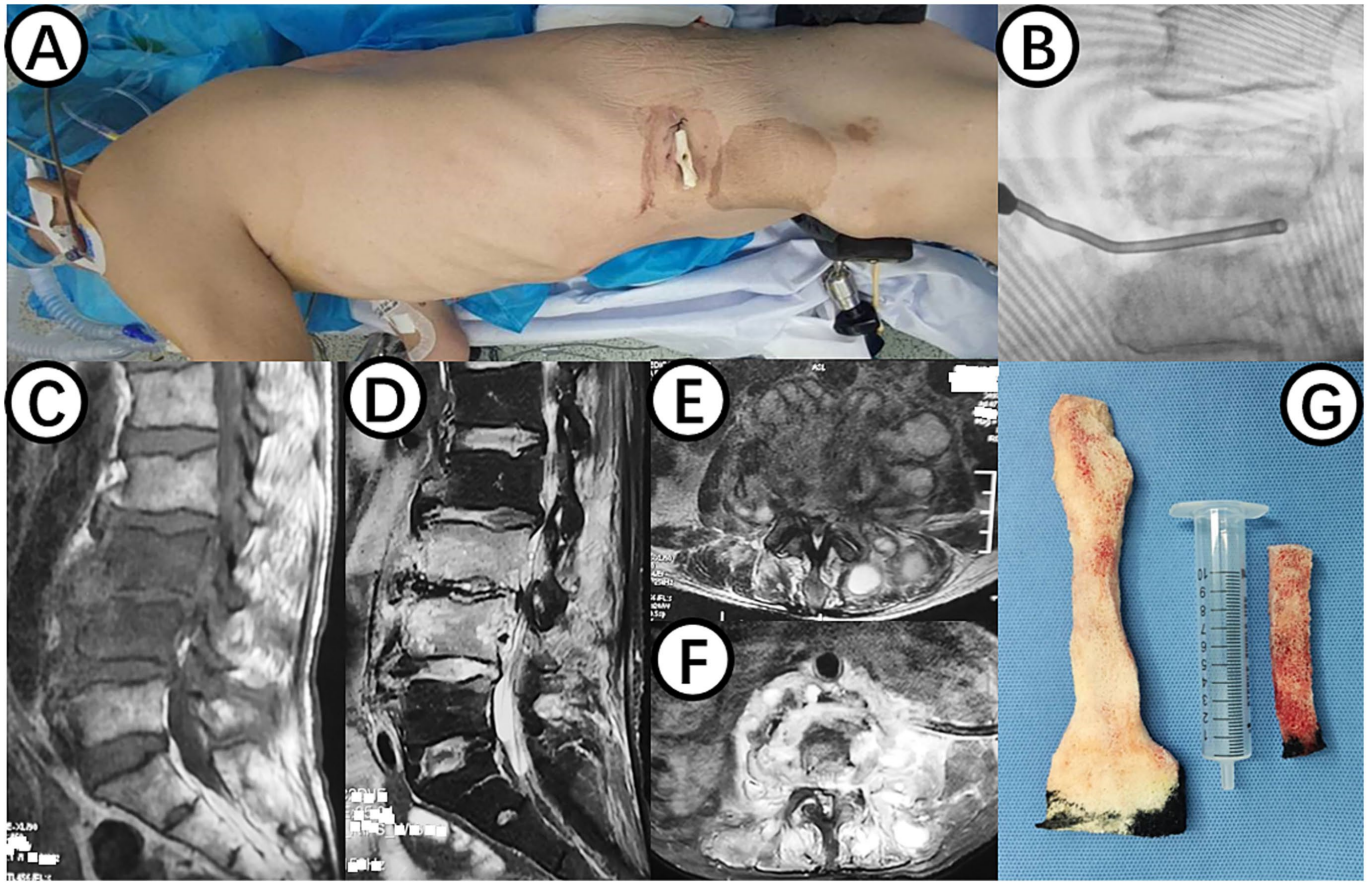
(A) Shows the surgical position for changing NPWT. (B) Displays intraoperative C-arm fluoroscopy positioning. (C,E) Present preoperative MRI scans showing high signal in the L3 and L4 vertebral bodies and intervertebral space, as well as abscess in the intervertebral space, bilateral lumbar muscles, and paraspinal muscles. (D,F) Demonstrate postoperative MRI showing decreased inflammation and edema signals in the L3 and L4 vertebral bodies and intervertebral space, with negative pressure sponge and drainage tube in place. (G) Depicts the NPWT sponge inside the body being replaced.
